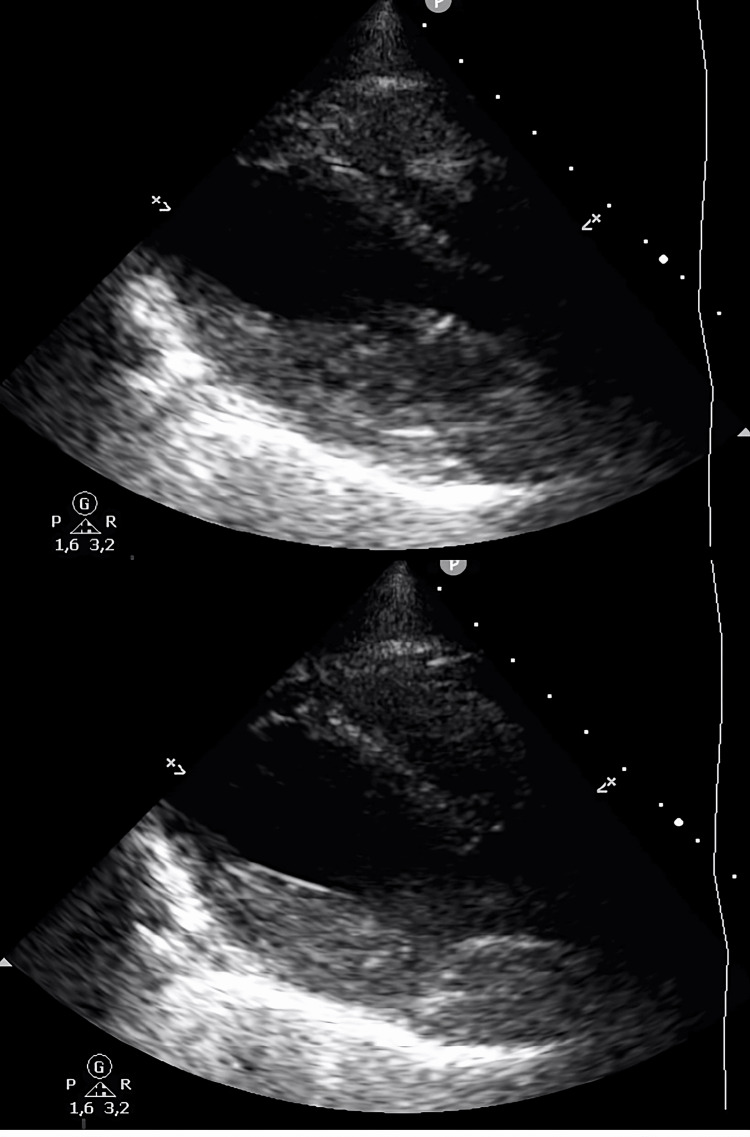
The first transthoracic echocardiography showed a left ventricle with hypokinesia of the mid-apical segments, in particular of the septum, with an ejection fraction of 38-40%.
